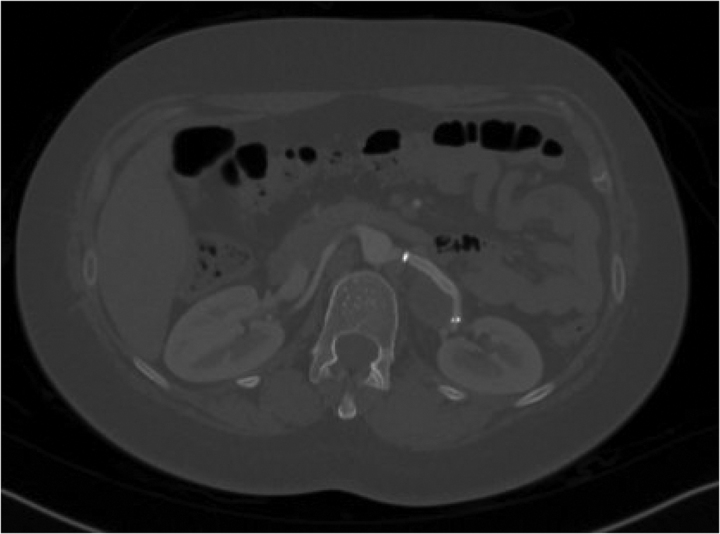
Postoperative computed tomography scan of the renal arteries axial view showed a post left renal artery aneurysm repair with a covered stent and a completely thrombosed aneurysm without extravasation or endoleak.
